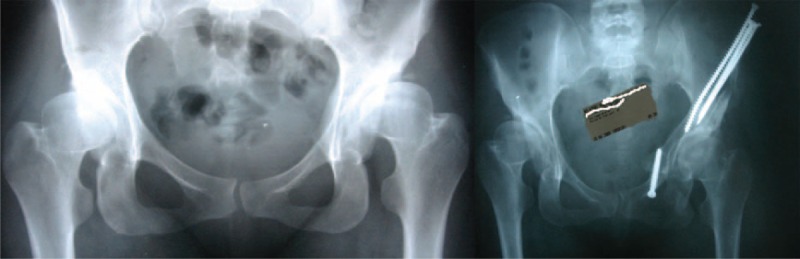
Patient 2: residual acetabular dysplasia; 30-year-old female; operated at the age of 1 for developmental dysplasia of hip; pre–postoperative radiography.
